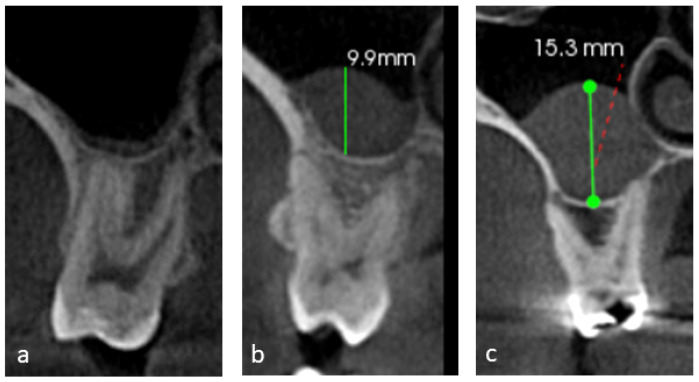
Coronal sections of CBCT images showing mucosal thickening grades. (a) Grade 1: 0–2 mm, (b) grade 2: 2–10 mm, and (c) grade 3: more than 10 mm.
